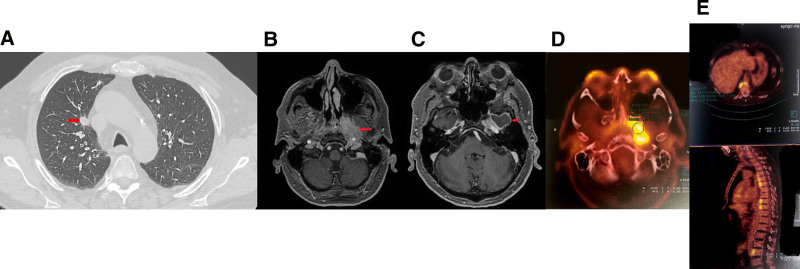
Pretreatment imaging assessment: (A) thoracic computed tomography (CT)-scan showing a speculated pulmonary nodule (red narrow); (B, C) cranial magnetic resonance imaging (MRI) showing a mass infiltrating the left infratemporal fossa (B) and pachymeningitis (C); (D, E) positron emission tomography (PET) scan showing hyperfixation of the ear, nose and throat (ENT) tumor (D) and the anterior longitudinal vertebral ligament (E).
